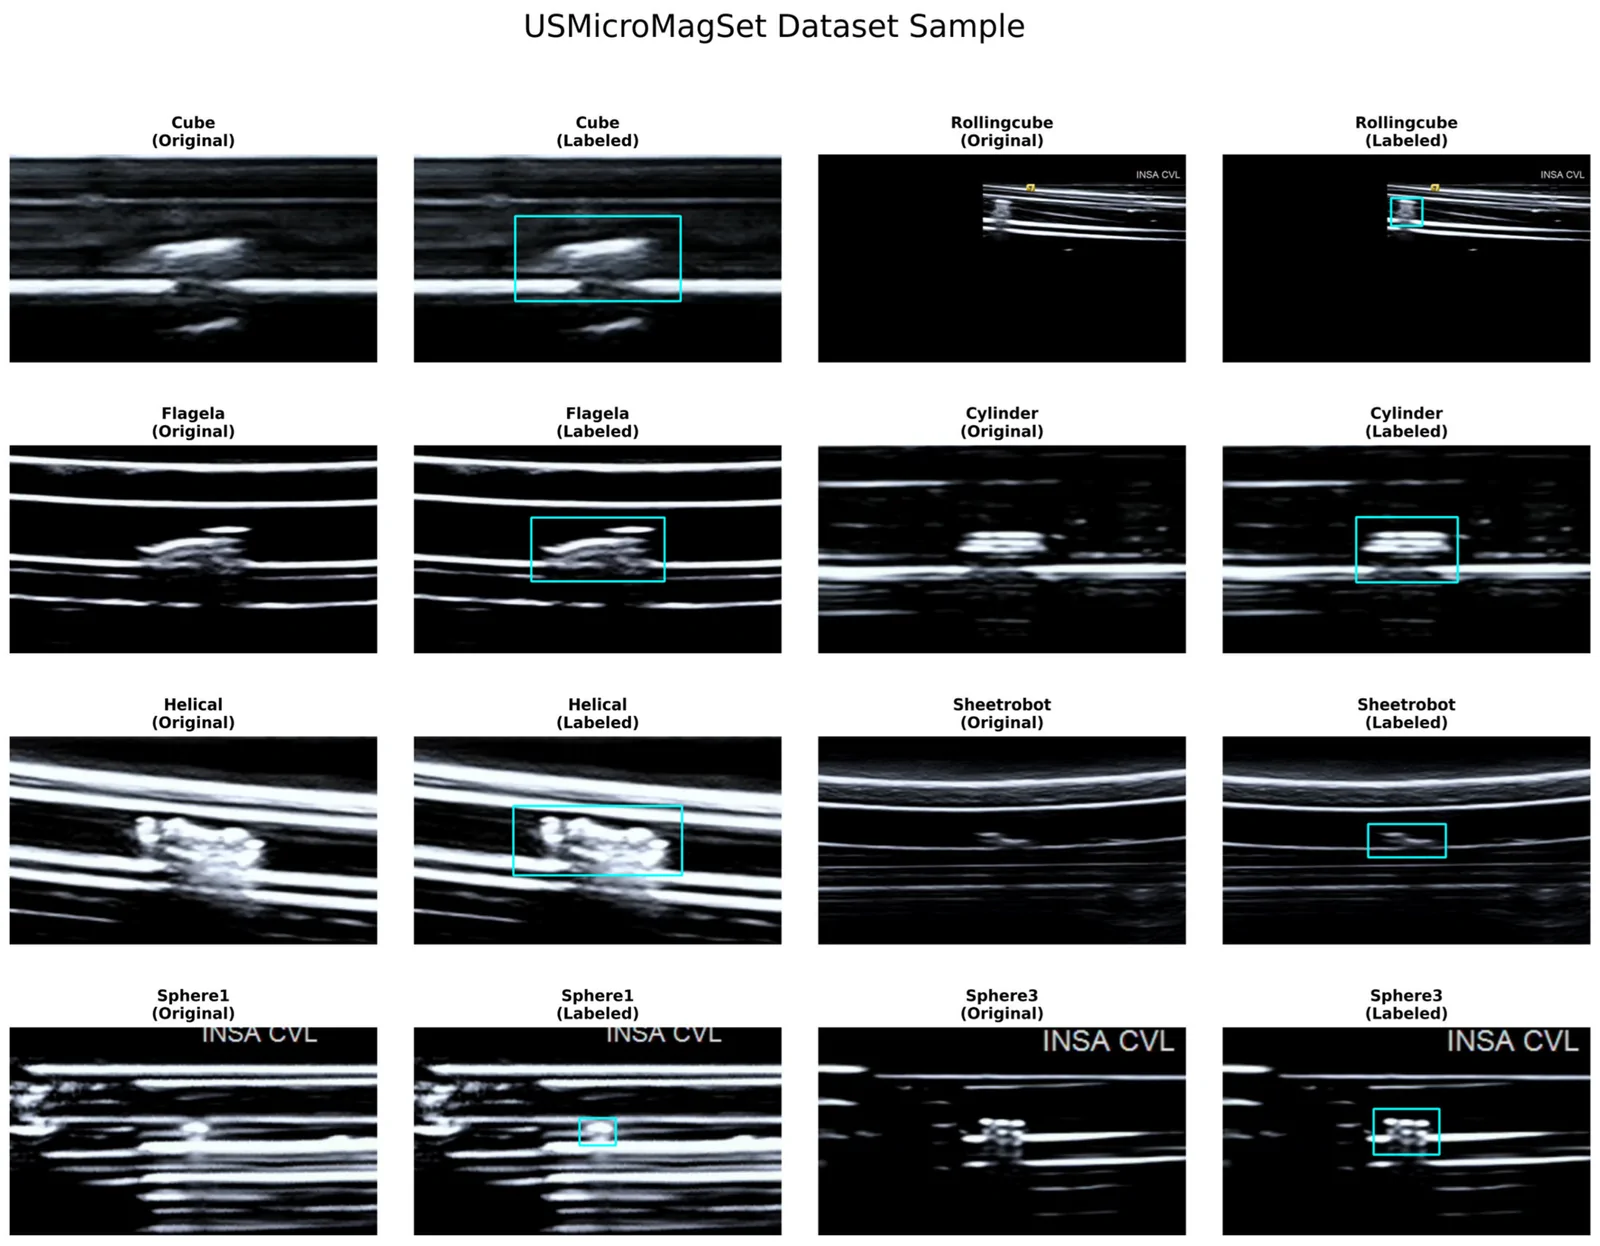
Sample of each microrobot ultrasound frame with unlabeled and labeled images with bounding boxes.
